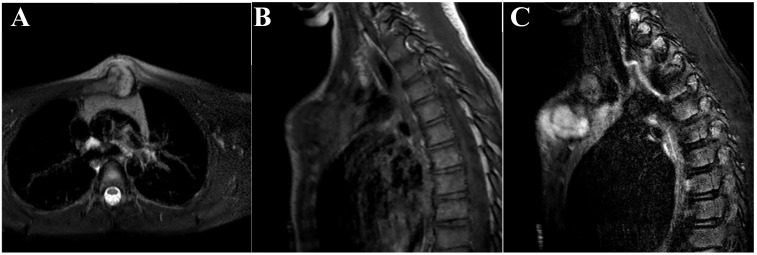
MRI scan of the chest after biopsy. (A) T2 weighted axial slice, (B) T1 weighted sagittal slice, and (C) short tau inversion recovery (STIR) sagittal slice of the chest.
